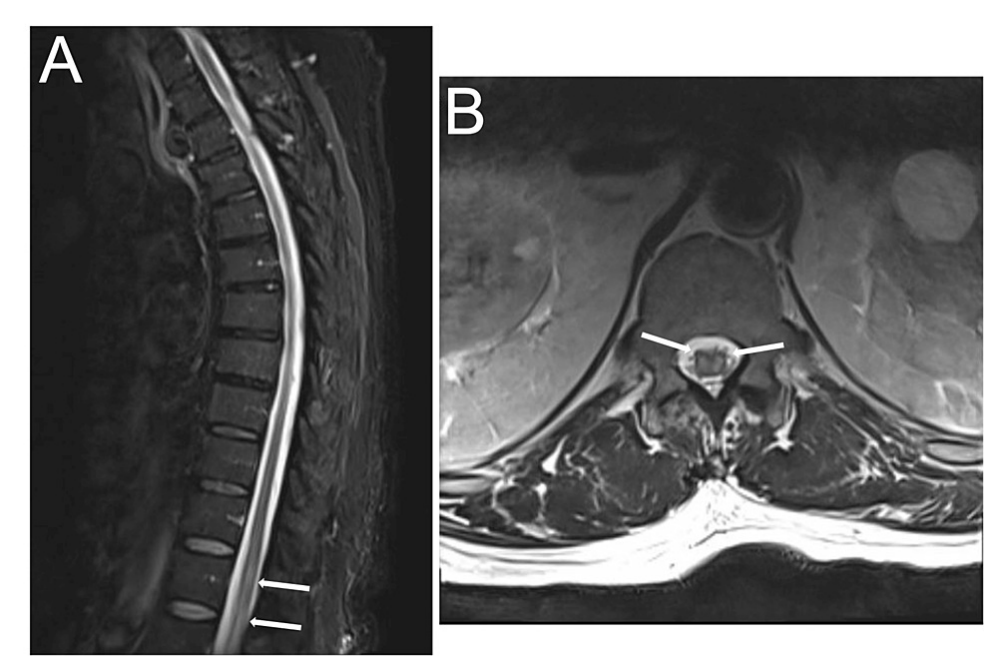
Follow-up MRI: magnetic resonance imaging sagittal (panel A) and axial (panel B) slices two weeks following discharge showing edema and expansion of the distal spinal cord with an abnormal signal on diffusion-weighted imaging concerning for ischemia of the conus medullaris (white arrows).
